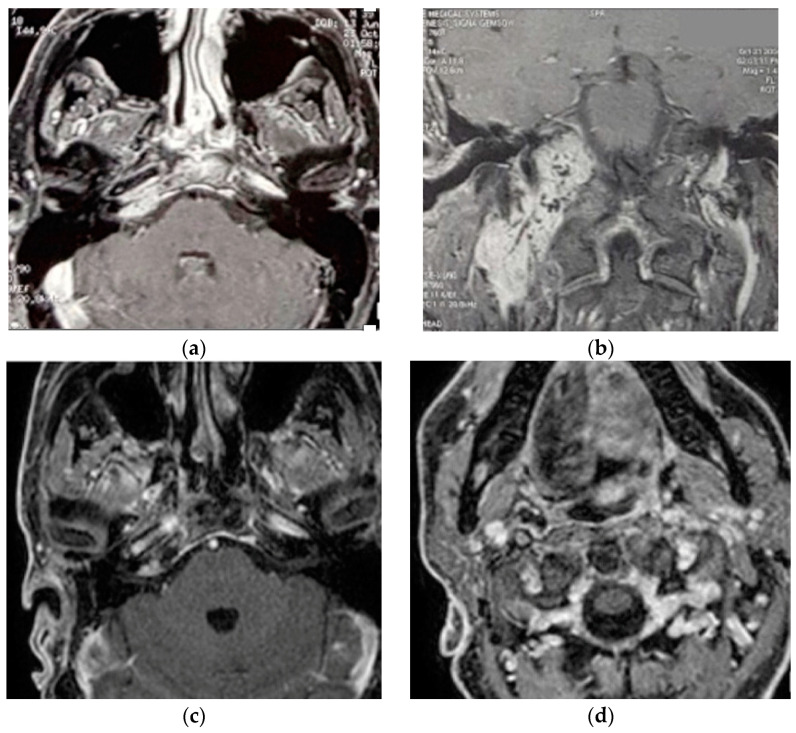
(Case 2) (a,b) Enhanced-axial T1W MRI showing C3Di1 + stage I VP. (c,d) Postoperative axial and coronal MRI showing no residual tumor.
